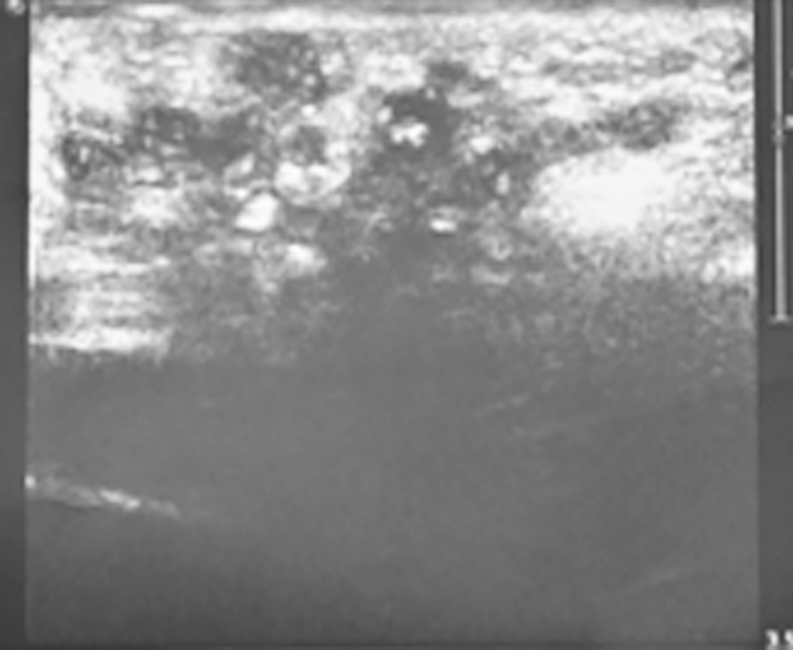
Ultrasound showing typical eumycetoma lesion; multiple thick walled cavities with sharp hyper-reflective echoes denoting grains without acoustic enhancement.
